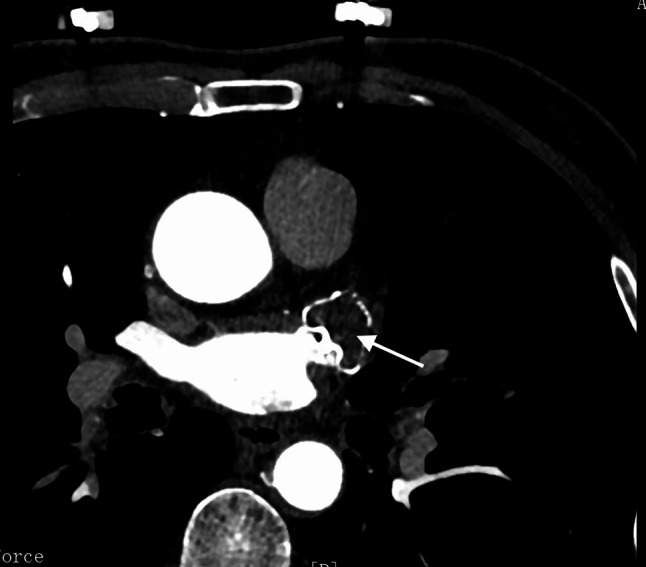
One case (a 72-year-old man with persistent atrial fibrillation) for CDE at 6 weeks follow-up after LAAC. LAA CTA showed no residual contrast agent flow inside the LAA (as shown by arrow)
